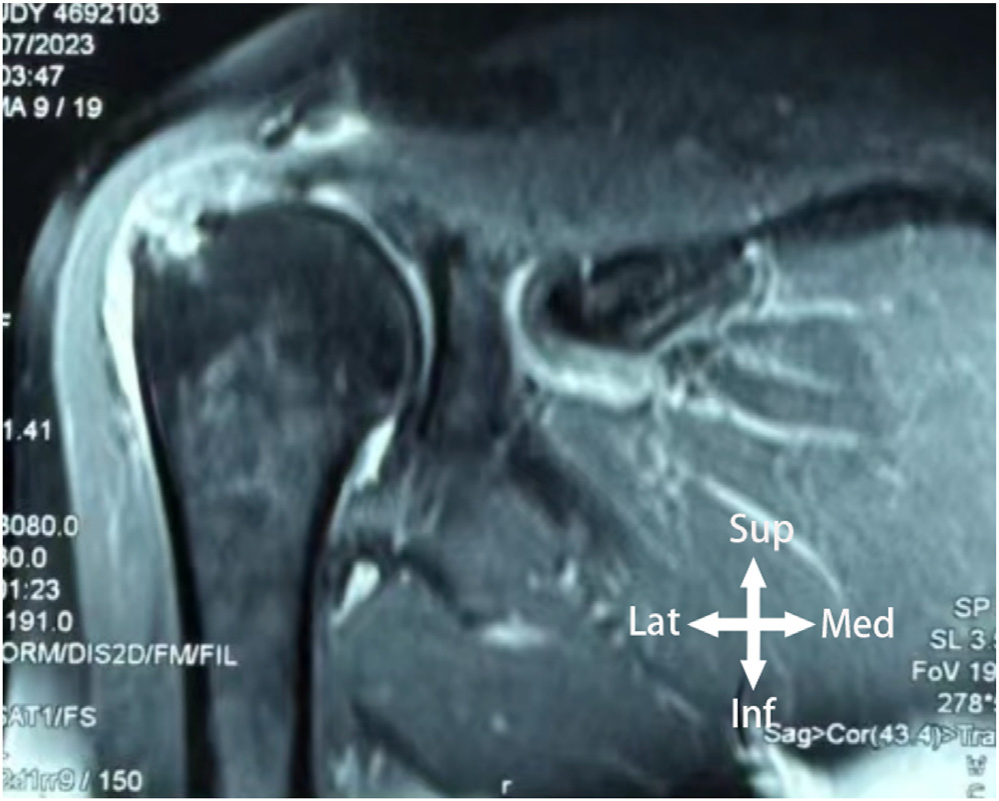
The patient’s magnetic resonance imaging reveals a rotator cuff injury with delaminated tears on the superior and inferior surfaces of the supraspinatus muscle.
